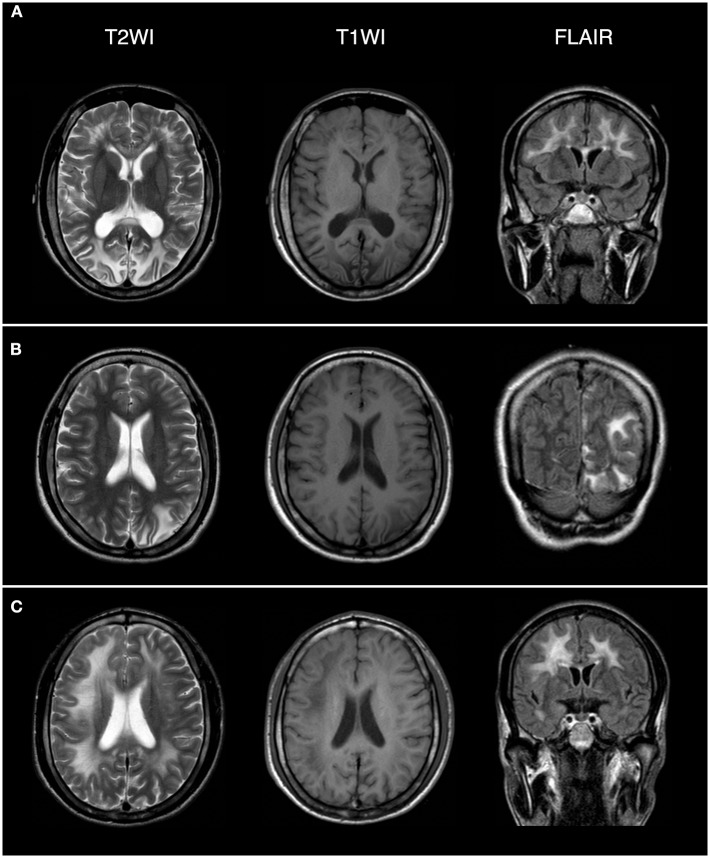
Brain magnetic resonance imaging (MRI) revealed extensive lesions in the white matter and subcortex in the proband (C), his oldest sister (A), and second oldest sister (B). The field intensity of MRI is 1.5T. The left side of the picture corresponds to the right side of the human body.
